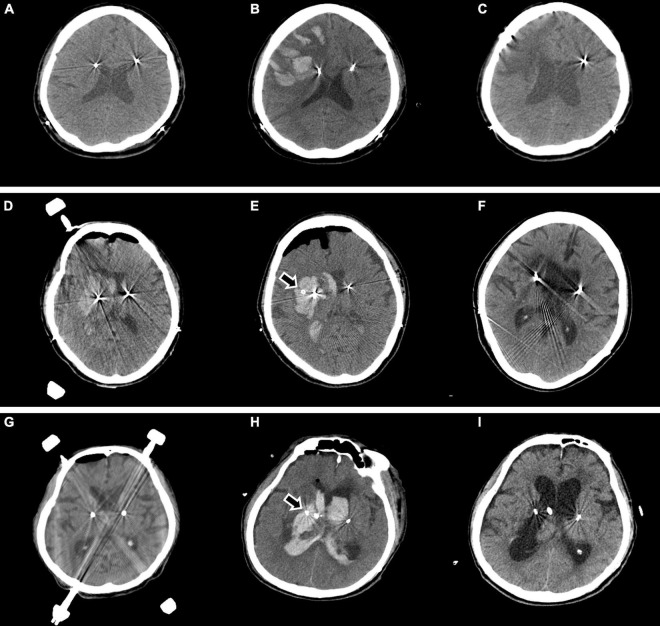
Major ICH requiring surgical treatment is observed in three cases. In one patient, no abnormalities are observed on brain CT immediately after surgery (A), but ICH developed in the right frontal lobe with seizures 8 h after surgery (B). The patient underwent craniotomy and hematoma evacuation due to a large ICH, increased edema, and a midline shift. Two months later, the patient was transferred for rehabilitation (C). In another patient, right thalamic ICH and IVH are observed on brain CT after intracranial electrode insertion (D), and hematoma catheter insertion was performed immediately (E arrow). After 1 month, the ICH was resolved, and the patient was transferred for rehabilitation (F). In another patient, no abnormalities are observed on brain CT immediately after surgery (G), but right thalamic ICH and IVH are observed on brain CT 16 h after the surgery, and hematoma catheter insertion was performed (H arrow). The hematoma is resolved (I), but the patient died from aspiration pneumonia on postoperative day 30. ICH, intracerebral hemorrhage; CT, computed tomography; IVH, intraventricular hemorrhage.
